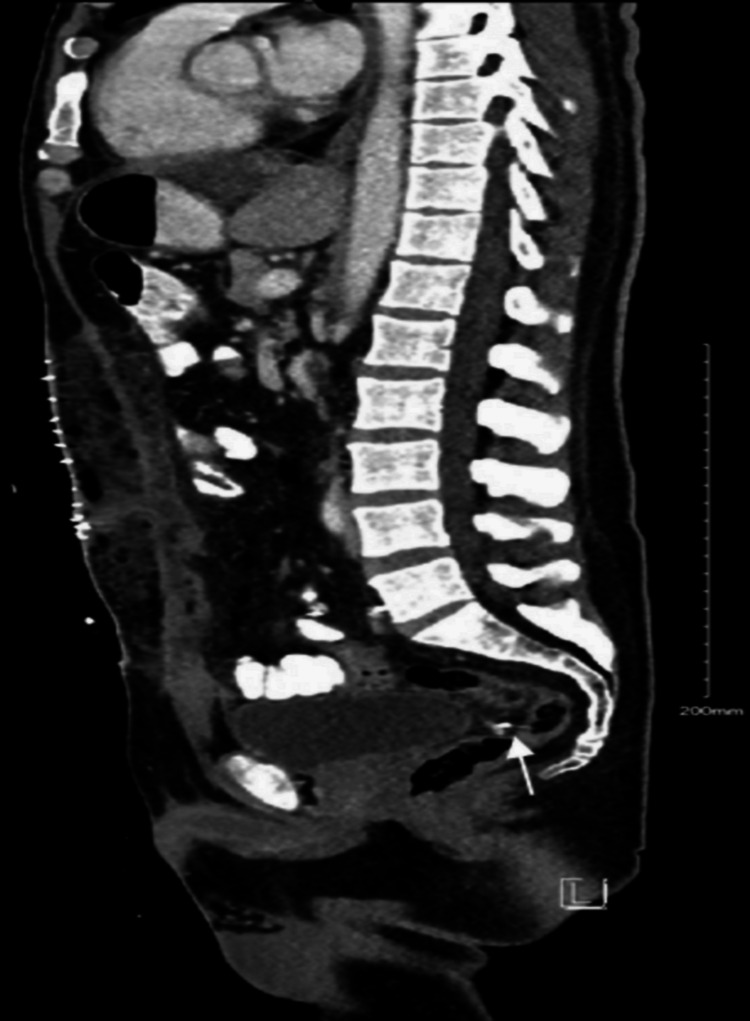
Enhanced sagittal CT scan of the abdomen and pelvis. An enhanced CT scan of the abdomen and pelvis revealed the patient to have alleviated large bowel obstruction following Hartmann’s procedure.
